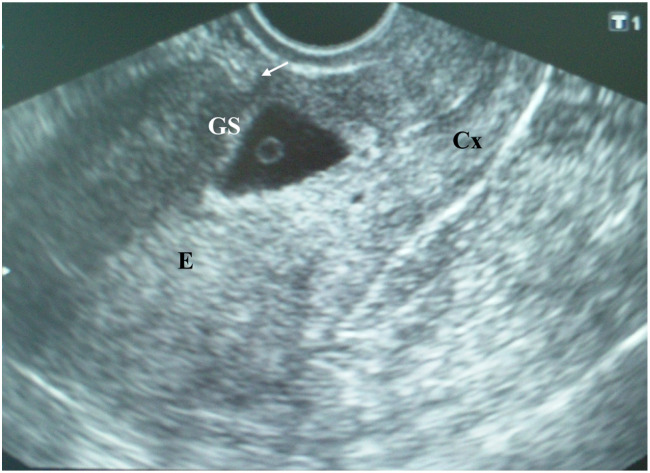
Transvaginal ultrasound examination of a cesarean scar pregnancy (CSP) at the sixth postmenstrual week showing an empty uterine cavity with a 9 mm endometrial strip (E) and a triangular gestational sac (10 mm in diameter) located within the isthmic part of the anterior uterine wall filling the niche of the scar, with a yolk sac inside covered with a thin myometrial layer 2 mm in diameter (arrow); the cervical channel (Cx) is empty; according to the presence of cross over sign (COS), this gestational sac could be identified as COS-1; according to the implantation of the gestation sac it is implanted in the niche of the scar (ultrasound sign reported by Kaelin Agten et al.); according to the position of the center of the gestational sac it could be classified as implantation bellow the uterine midline (classification proposed by Timor-Tritsch et al.) (explanation in *Discussion*).
